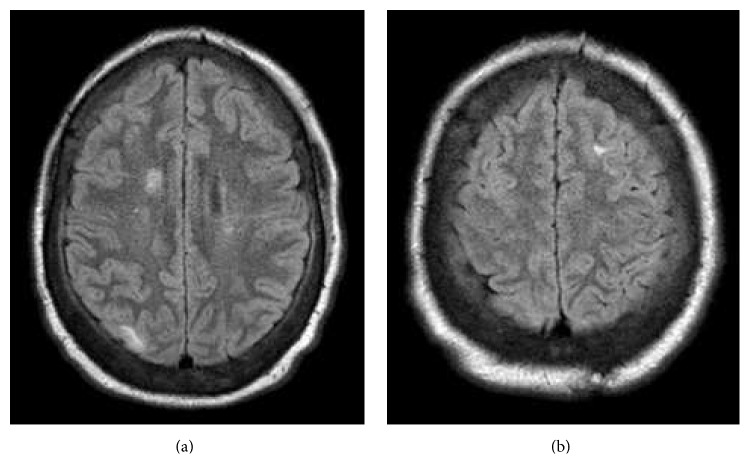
T2 FLAIR axial MRI showing subcortical areas of mixed signal mass in the right occipital cortex (a) and left frontal cortex (b), suggestive of cortical tubers.
